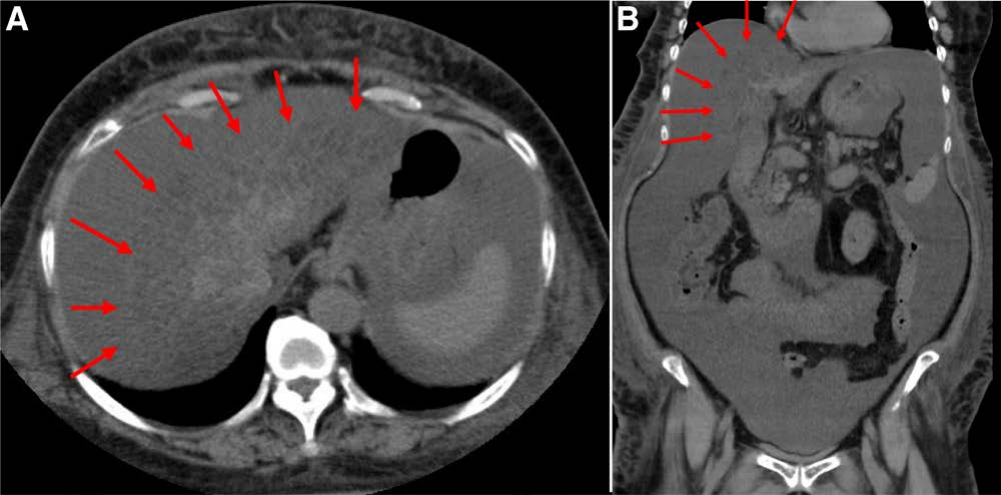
Computed tomography (CT) scan was taken during the emergency hospital visit on February 22, year X. (A) Horizontal and (B) vertical sections are shown. Arrows show the liver margins. The liver had severe atrophy and fatty changes with a mottled pattern. In addition, a large amount of ascites was visible in the abdominal cavity. X = year of the patient’s death.
